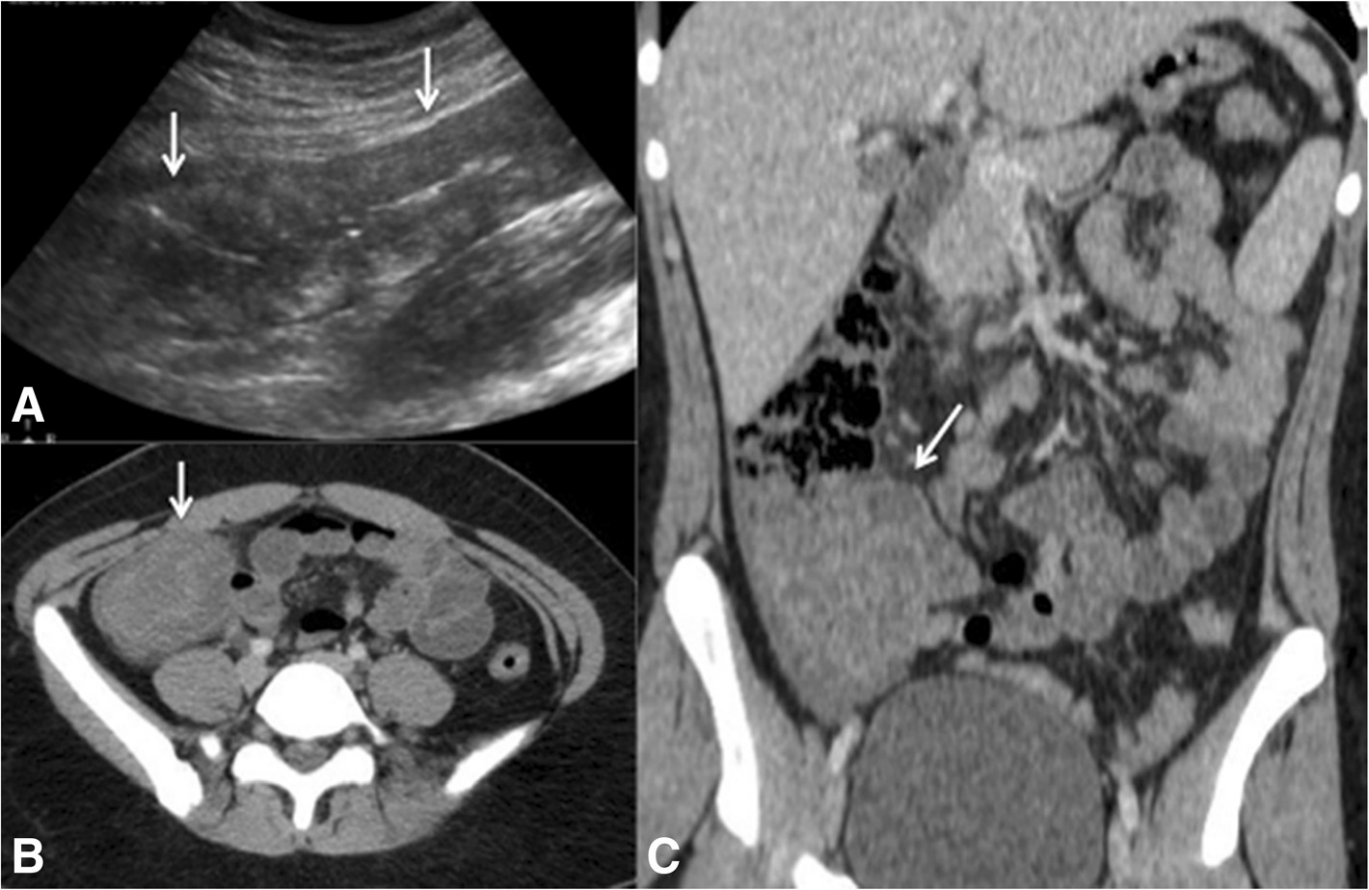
A 16-year-old boy with neutropenic typhlitis. Patient under treatment for leukemia, presented with abdominal pain in the right iliac fossa. (A) Ultrasound image showing diffuse thickening of the walls of the cecum and ascending colon (arrows). (B) Axial and (C) coronal computed tomographic slices obtained after intravenous contrast administration confirmed the ultrasound findings, showing thickening of the cecum wall and proximal portion of the ascending colon (arrows), compatible with typhlitis.
